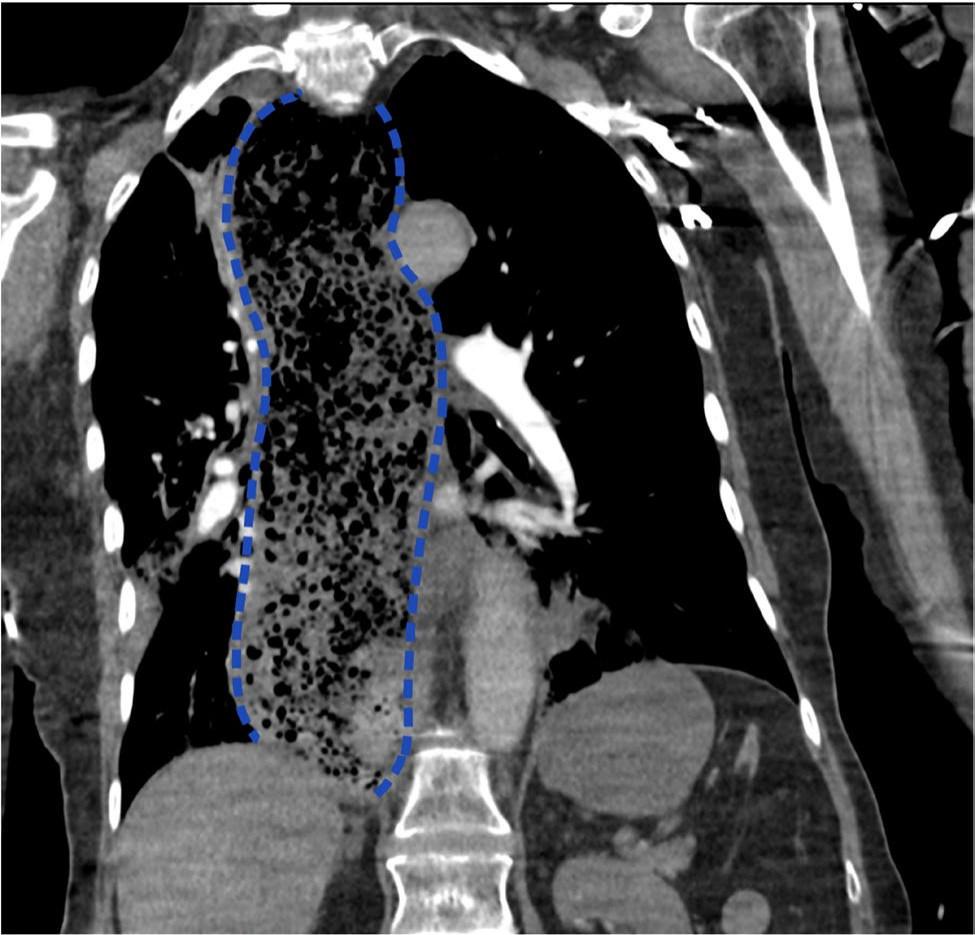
Coronal view of CT pulmonary angiogram taken on admission showing a grossly dilated oesophagus with extensive food residue, highlighted in blue
